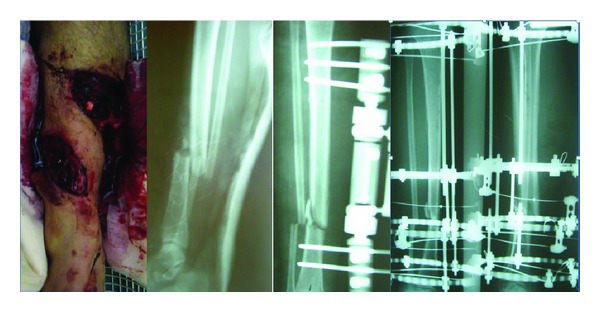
Case 7: AO/OTA: 42B2, GA: 3B, 48-year-old male, traffic accident, elective surgery (third stage): Ilizarov, 10-month followup, X-ray: there is no adequate callus.
